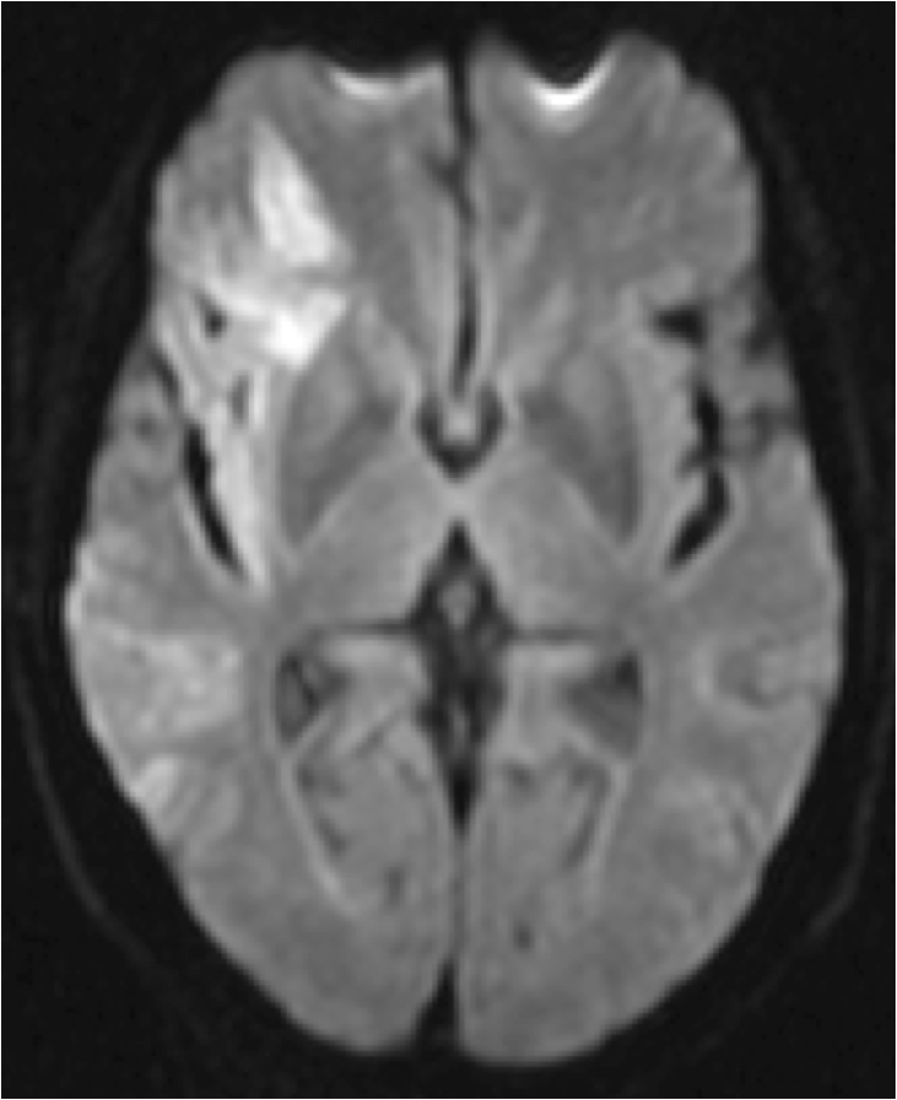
Diffusion-weighted brain MRI of patient who had left ventricular thrombus formation. The scan demonstrates an ischemic stroke in the territory of the right middle cerebral artery
